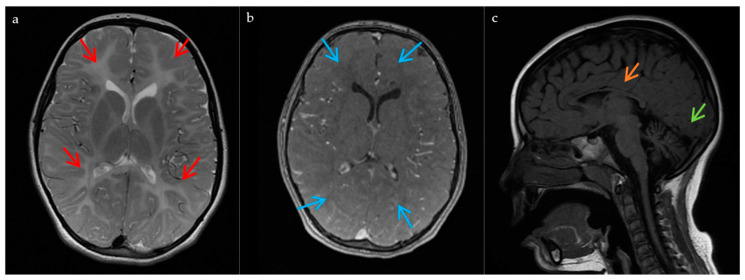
MRI findings in the patient. These images, obtained at the age of 7, show: (a) Hypomyelination process with diffuse hyperintensity of the supratentorial and cerebellar white matter on the T2-weighted images (red arrows); (b) T1 diffuse isointensity of the supratentorial white matter on the T1 sequences (blue arrows); (c) Cerebellar atrophy (green arrow), and a thinned corpus callosum (orange arrow).
